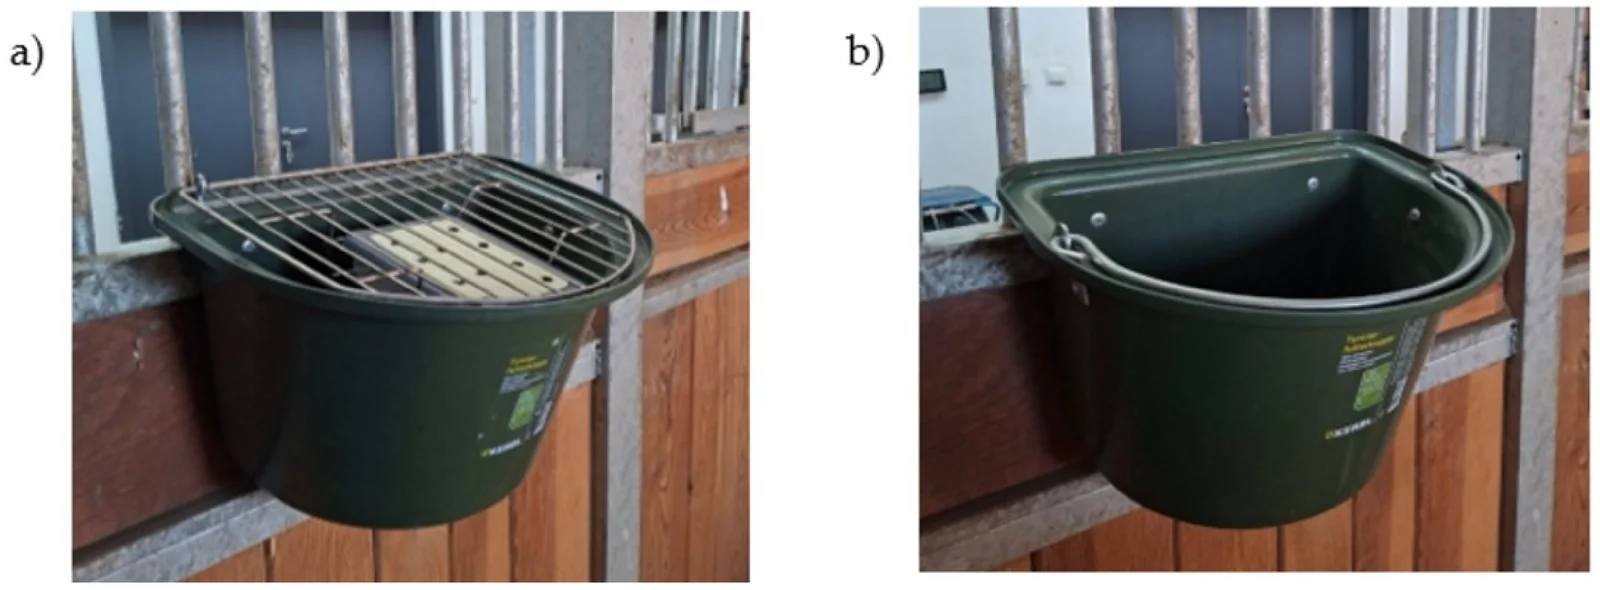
The ST crib (a) and CT crib (b) used in the experiment. ST—crib used in the smelling test, CT—crib used in the consumption test.
